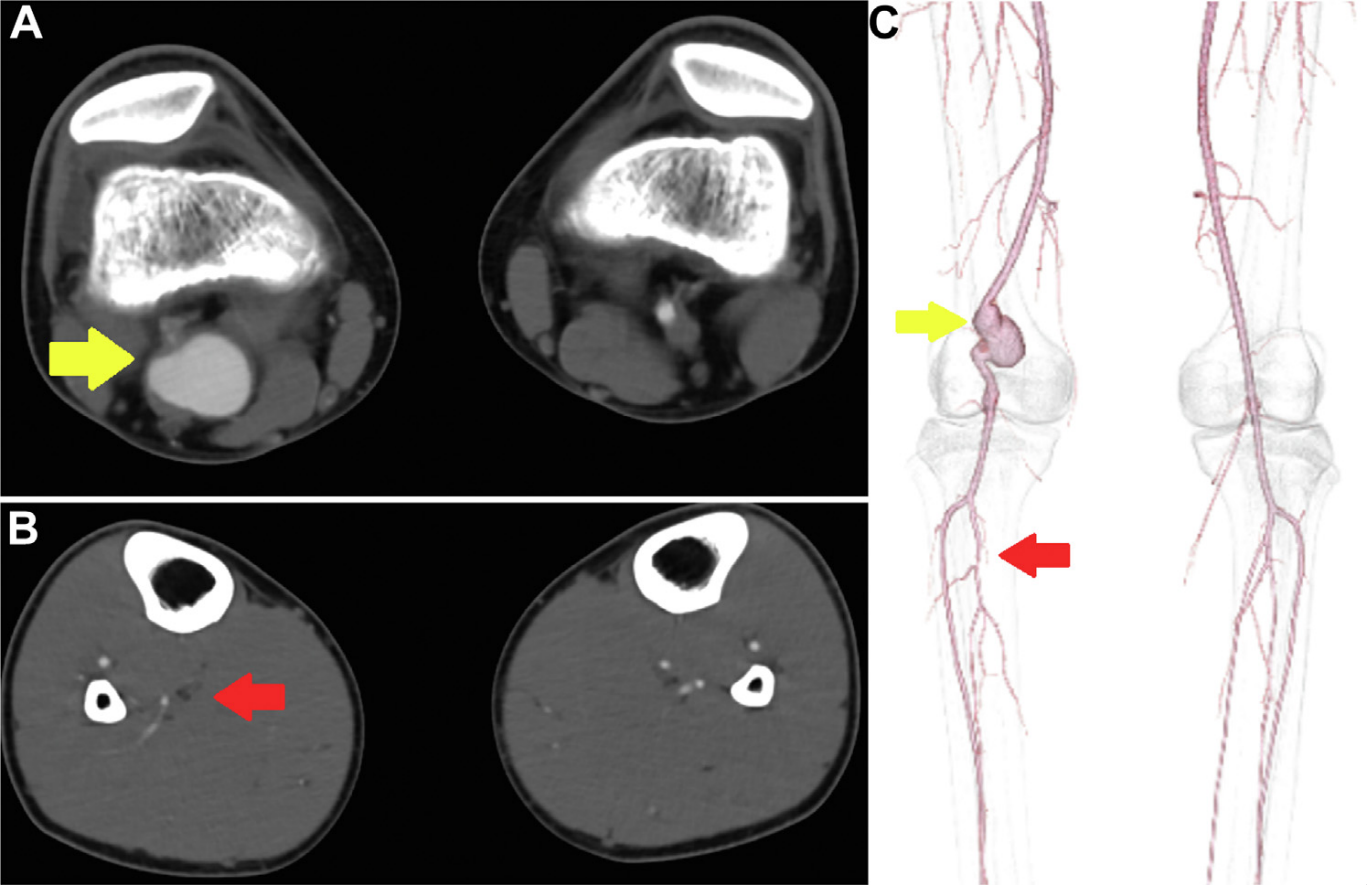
Preoperative computed tomography (CT) findings. A, Right popliteal aneurysm (*yellow arrow*) 47 × 33 × 27 mm in size. B, Occlusion of the right posterior tibial artery (*red arrow*). C, Three-dimensional computed tomography angiogram of the lower limb artery.
